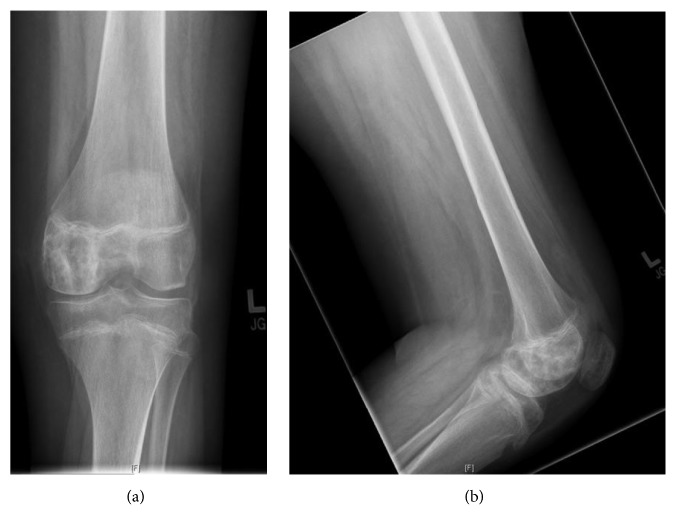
Anteroposterior (a) and lateral radiographs (b) of the left knee after core biopsy, September 2009.
